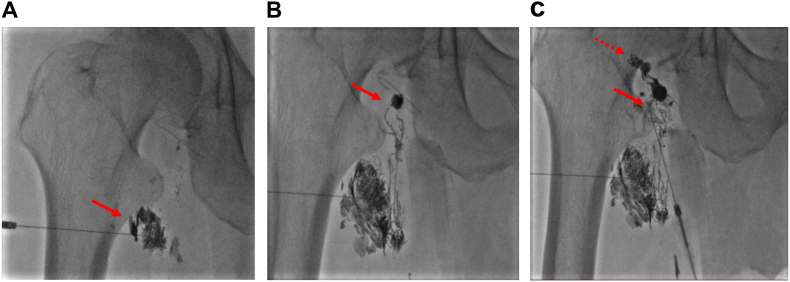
Fluoroscopic image revealing lymphangiography using lipiodol (A) The residual caudal right inguinal lymph node was punctured using a 23 G 60-mm needle under ultrasound guidance and lipiodol was injected (arrow). (B) Intranodal lymphangiography revealing lymphatic leakage from the lymphatic duct (arrow). Intranodal glue embolization was performed using the lipiodol–n-butyl-2 cyanoacrylate (NBCA) mixture. (C) Furthermore, the lymphatic duct was directly punctured under fluoroscopy (arrow). The cranial lymphatic leakage (dashed arrow) was observed and embolized using the lipiodol–NBCA mixture.
